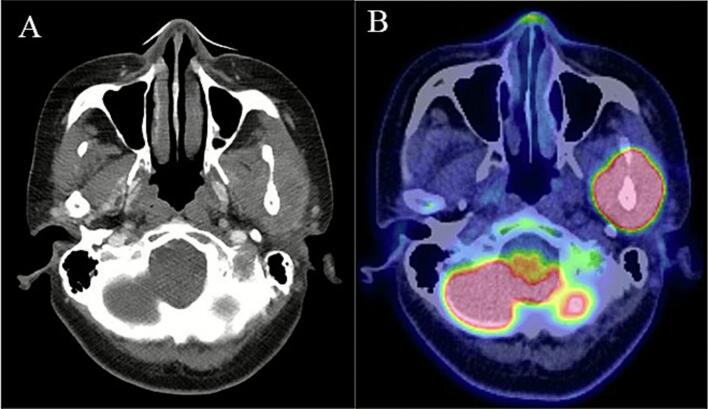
Pretreatment imaging findings A: Contrast-enhanced CT reveals a mass in the left mandible. B: PET/CT shows intense uptake corresponding to the left mandibular mass.
